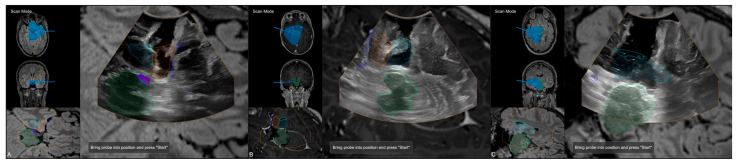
Navigated intraoperative ultrasound after resection of the amygdala (orange) and hippocampus (blue) visualizing the extent of resection and surgical trajectory in axial views (A,B) and oblique view (C).
